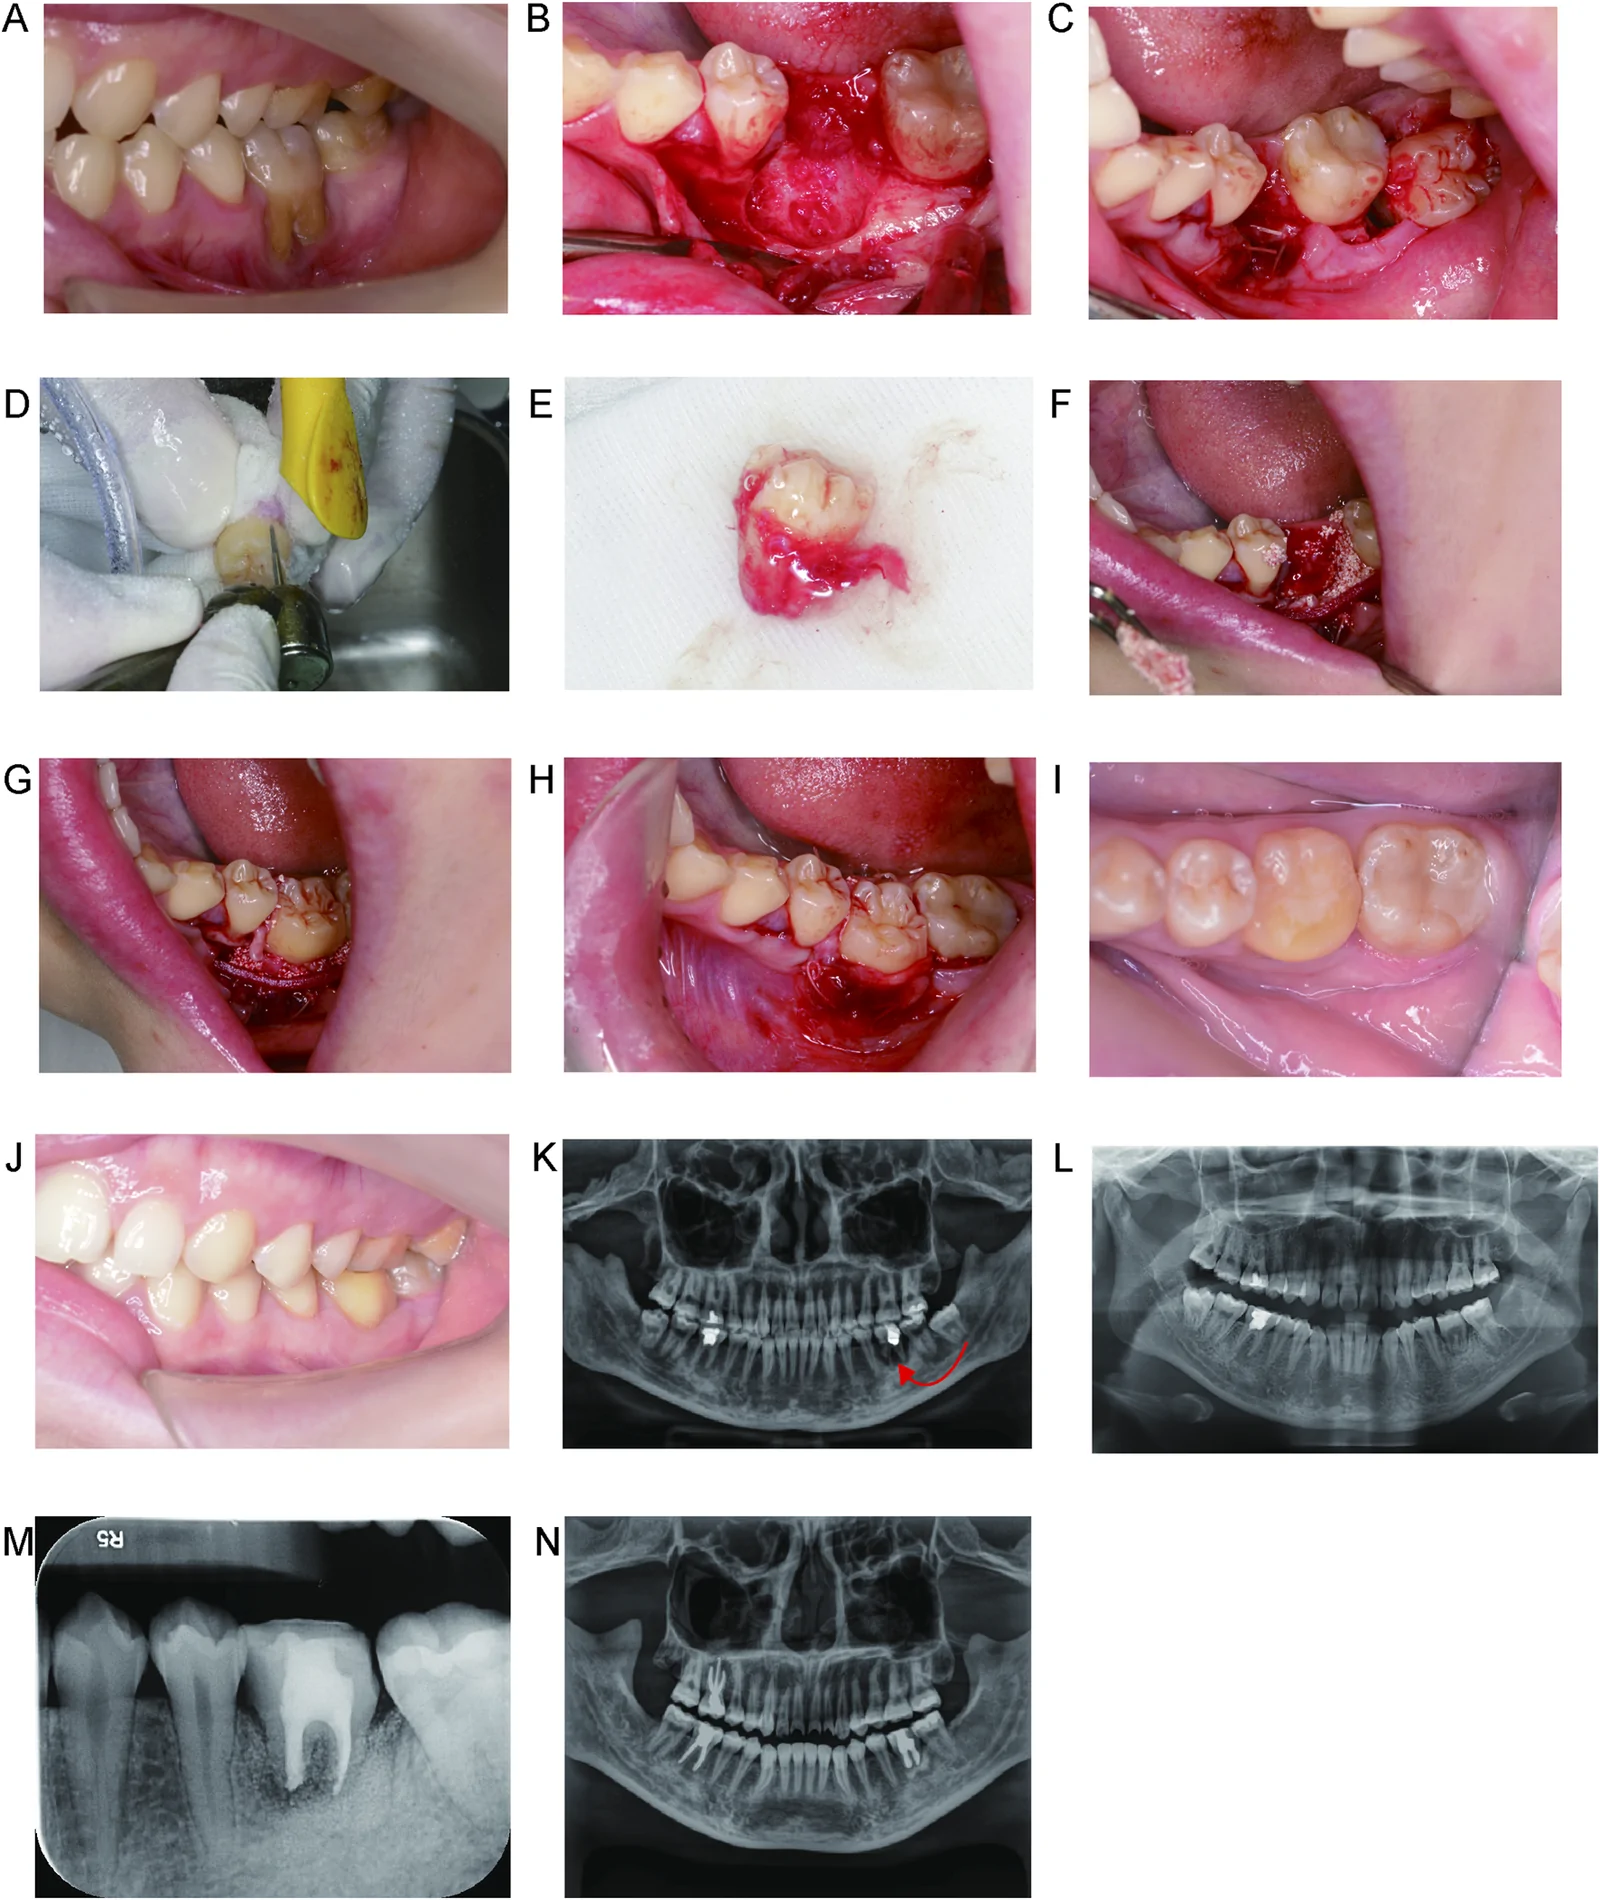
Clinical photographs and radiographic images of Case 2 showing autogenous tooth transplantation of tooth 38 to the tooth 36 recipient site. (A) Initial intraoral photograph of tooth 36. (B) Minimally traumatic extraction of tooth 36. (C) Minimally traumatic extraction of donor tooth 38. (D) Removal of calculus from the cervical region of tooth 38. (E) Photograph of the extracted donor tooth 38. (F) Placement of sticky bone into the prepared recipient socket at the tooth 36 site after socket refinement. (G) Insertion of tooth 38 into the prepared tooth 36 recipient socket. (H) Stabilization of transplanted tooth 38 at the tooth 36 site. (I,J) Intraoral photographs at the 1-year follow-up after autogenous tooth transplantation. (K) Preoperative CBCT image before autogenous tooth transplantation. (L) Immediate postoperative panoramic radiograph after transplantation. (M) Periapical radiograph after completion of root canal treatment 3 months after transplantation. (N) CBCT image at the 1-year follow-up. ATT, autogenous tooth transplantation; CBCT, cone-beam computed tomography.
